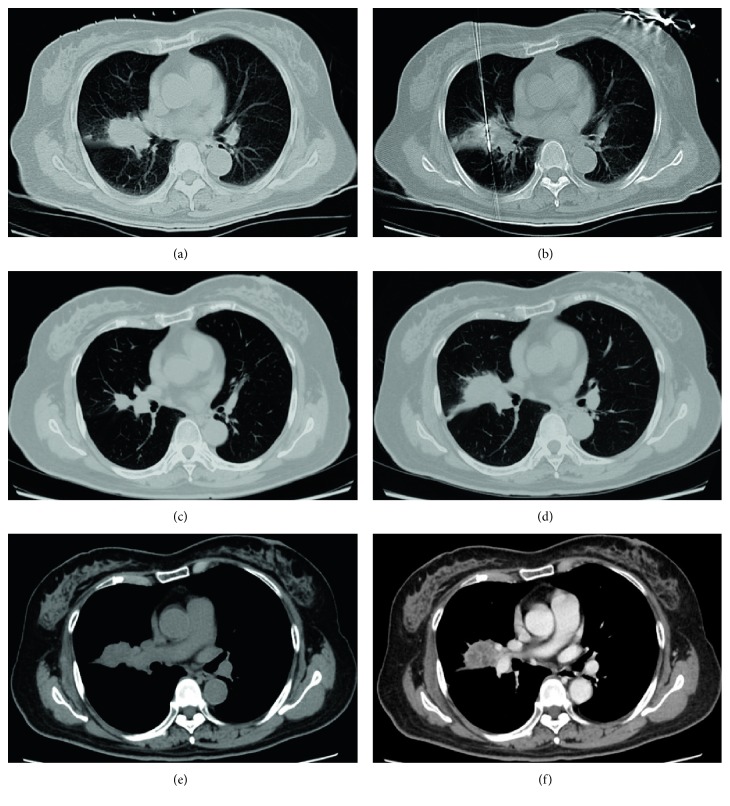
A 66-year-old female with nodular lung metastatic lesion with a diameter of 2.5 cm (a). Ablation was performed after accurate insertion of MWA probe into the focus of tumor (b). The lesion reduced obviously with some fiber cable around it on 3 months follow-up (c). However, the volume of the tumor increased again on 6 months follow-up ((d) lung window; (e) soft tissue window; (f) contrast-enhanced CT), which was consistent with the description of local tumor progression.
